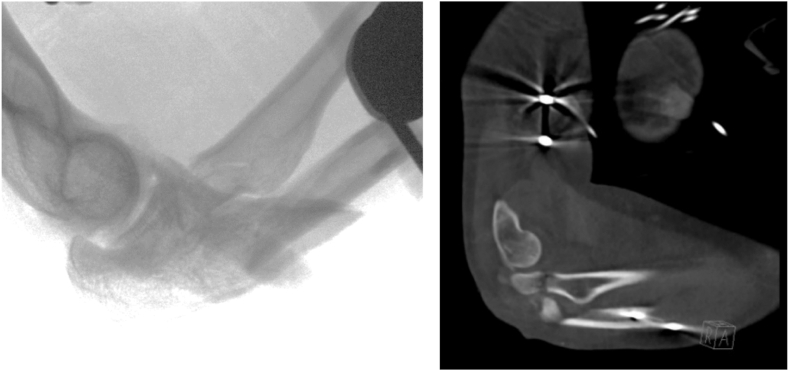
Imaging of the right elbow showing extensive comminuted fracture of the radial neck with displacement, comminuted fracture of the proximal ulna, and fracture of the coronoid process. Artefacts of the external fixator in the humerus are visible. Preoperative X-ray imaging not available due to the extent of other injuries.
